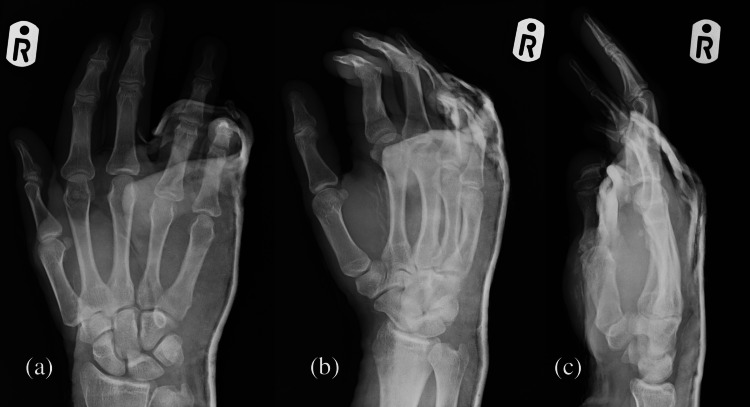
Post-manipulation check X-ray showing realignment of ring and little finger carpometacarpal joints. (a) Anteroposterior view, (b) oblique view, and (c) lateral view.
